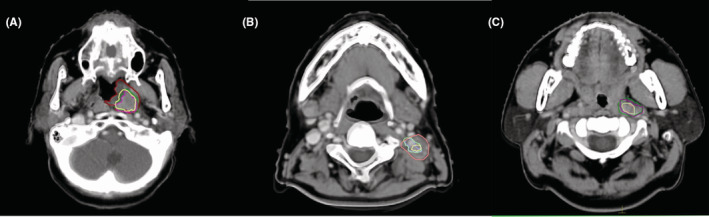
The primary tumor's gross tumor volume as well as that of the cervical and retropharyngeal lymph nodes on pretreatment CT and three repeat CTs. (A) Primary tumor: the red, yellow, green, rosy lines; (B) cervical lymph nodes: the orange, green, purple, yellow lines; (C) and retropharyngeal lymph nodes: the green, purple, pink, light green lines denote the gross tumor volumes on pretreatment CT and the first, second, and third repeat CTs, respectively. CT, computed tomography.
